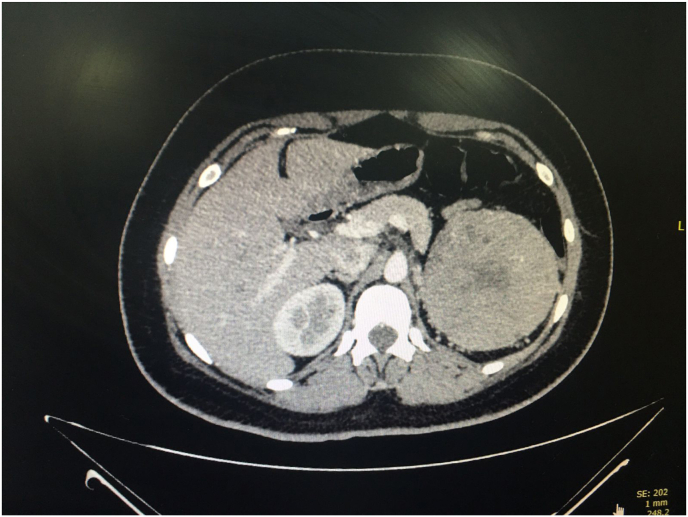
Contrast-enhanced computed tomography (CT) scan demonstrating a well-defined, mildly enhancing mass measuring 93 × 92 × 96 mm located in the upper pole of the left kidney (red arrow).
